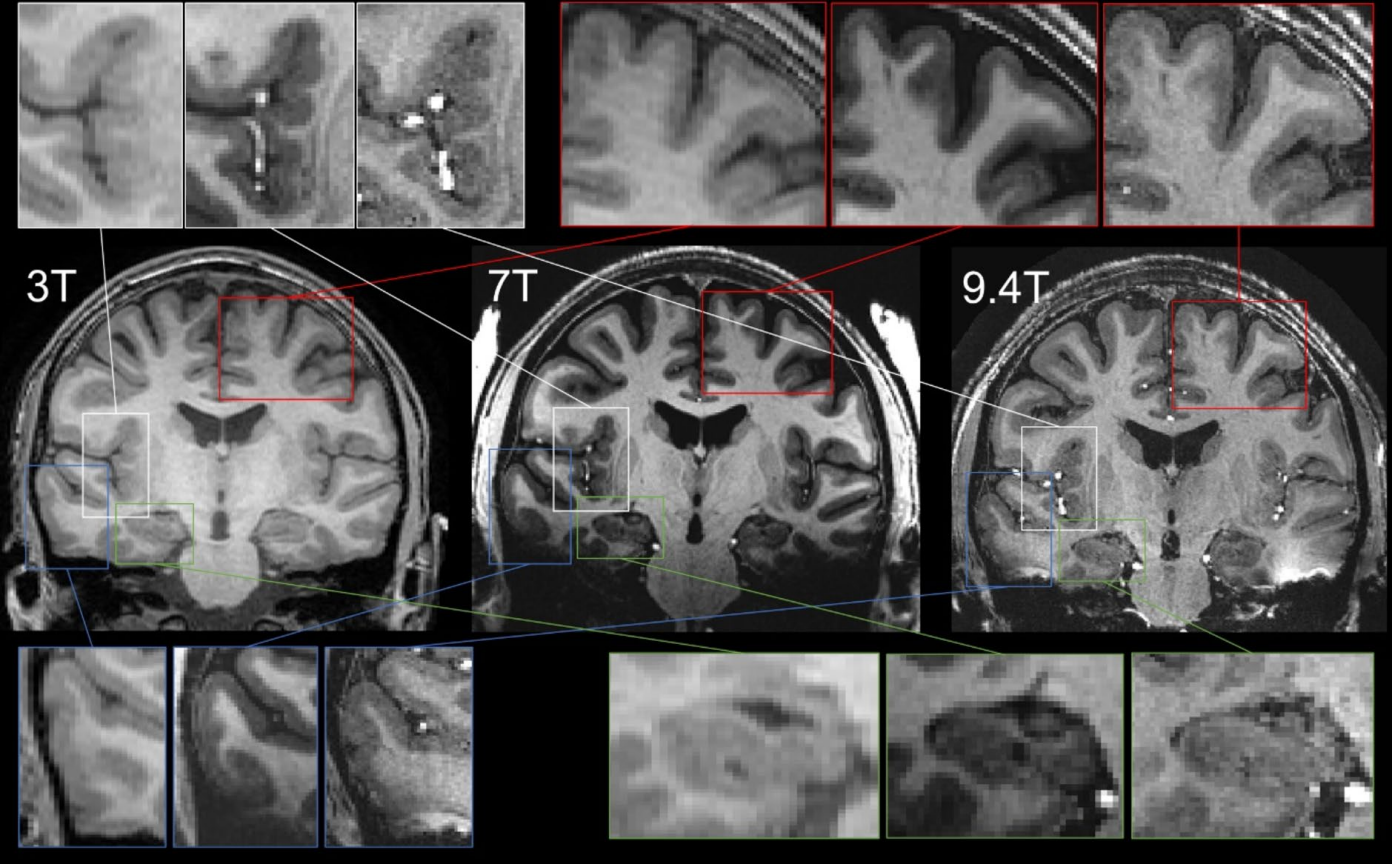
Coronal view of the 3T, 7T and 9.4T images, showing corresponding magnification of regions of interest in MRI epilepsy evaluation with top left the insular area, top right grey-white matter junction in the frontal area, bottom left lateral temporal area, bottom right hippocampus
